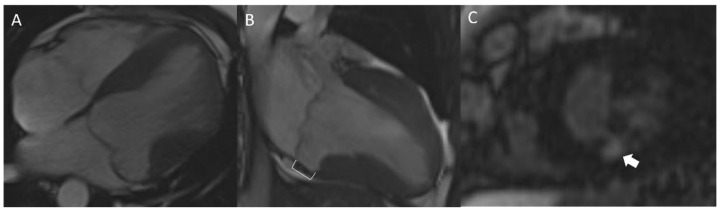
Cardiac magnetic resonance findings of arrhythmic mitral valve prolapse and mitral annular disjunction. (A) Bileaflet prolapse seen in a view analogous to apical 4 chamber view. (B) Mitral annular disjunction (bracket) where the mitral valve hinge point separates from the basal left ventricular myocardium and has systolic excursion into the left atrium. (C) Late gadolinium enhancement of a papillary muscle (arrow) seen in a view analogous to parasternal short axis.
